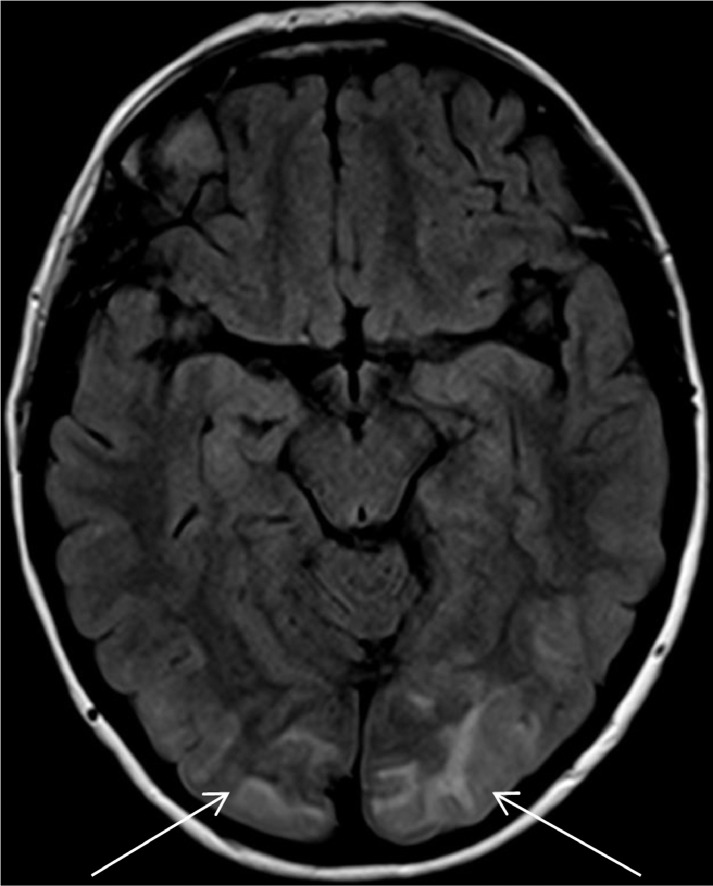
Axial FLAIR MRI brain demonstrating high signal within the subcortical white matter of both occipital lobes.
